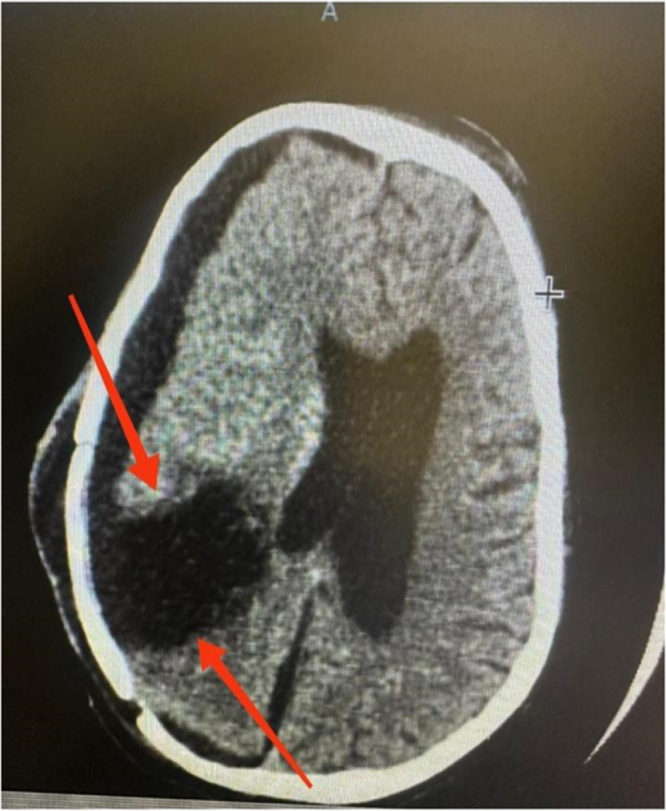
Postoperative MRI demonstrating gross total resection of the intracranial lesion with postoperative changes in the surrounding parenchyma.
